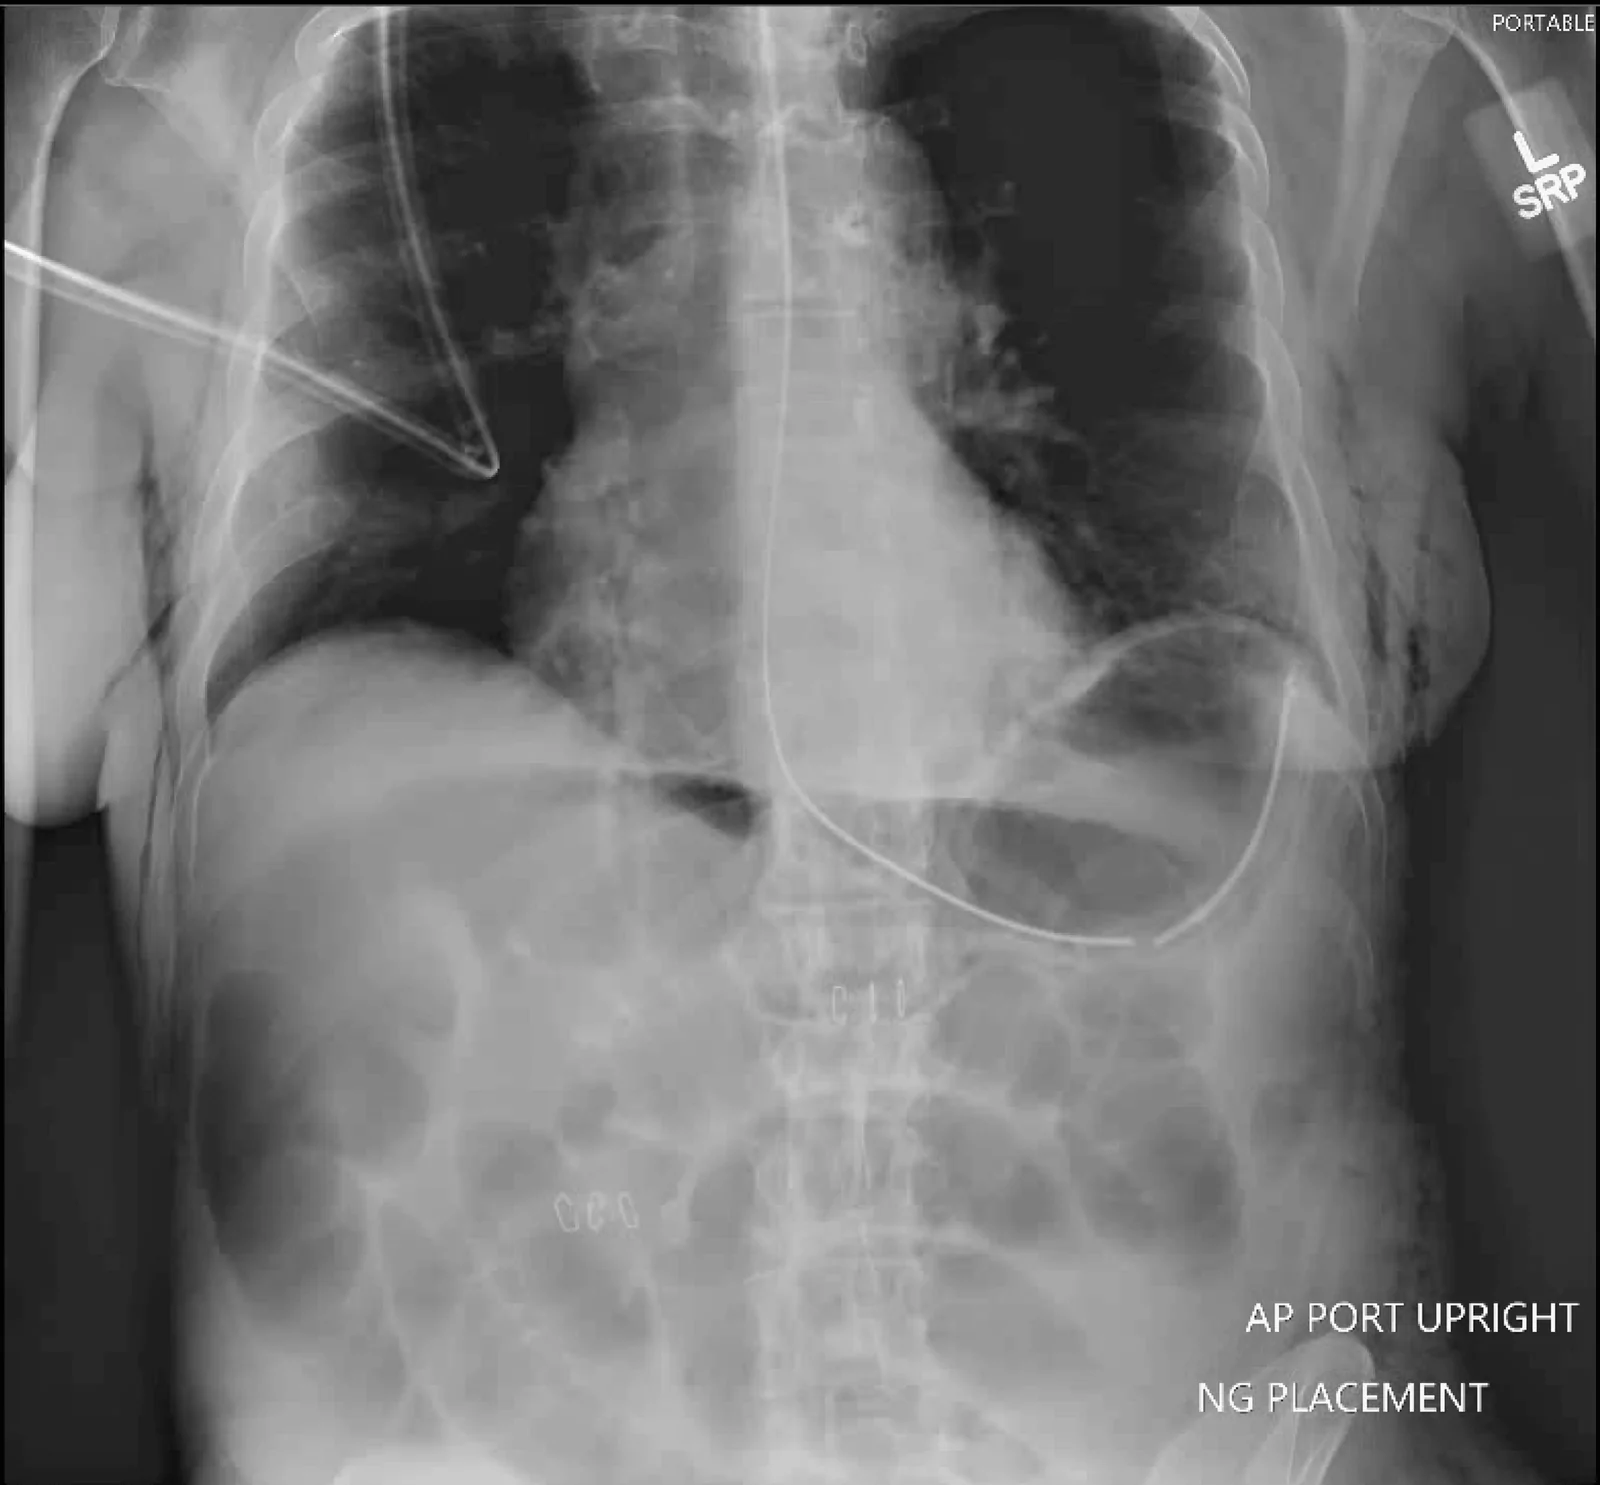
Abdominal radiograph The image demonstrates diffuse gaseous bowel distention consistent with postoperative ileus two months after the onset of symptoms
